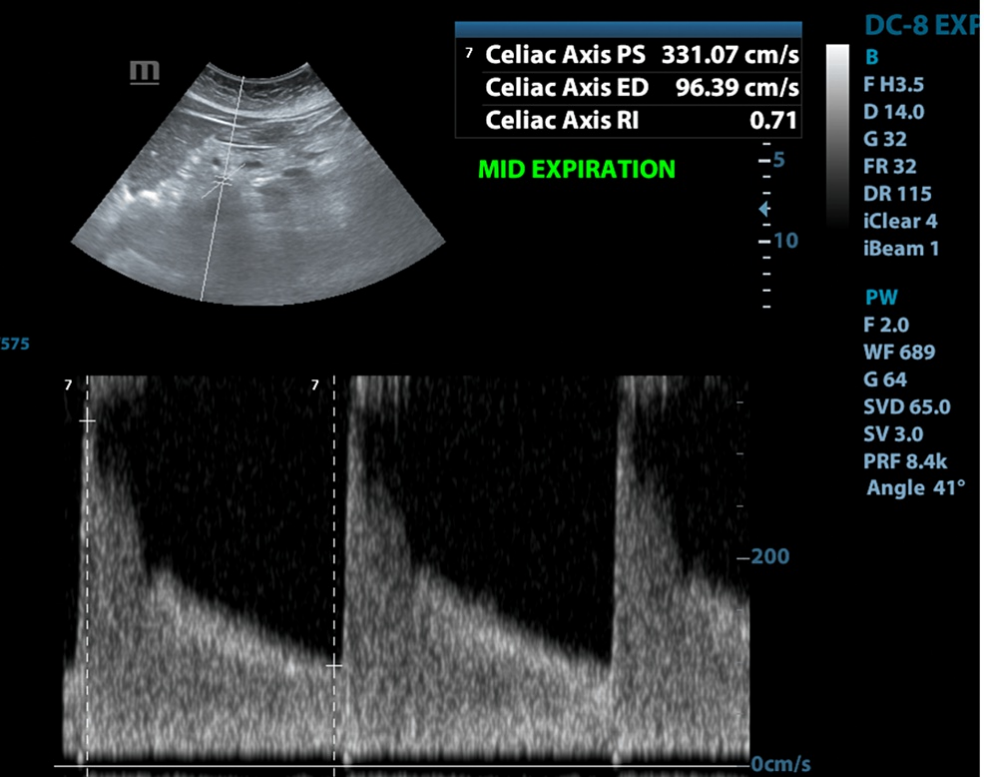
Spectral Doppler ultrasound in the mid-expiration over the compressed segment of the coeliac trunk demonstrating an elevated peak systolic velocity (PSV) of 331.07 cm/s
